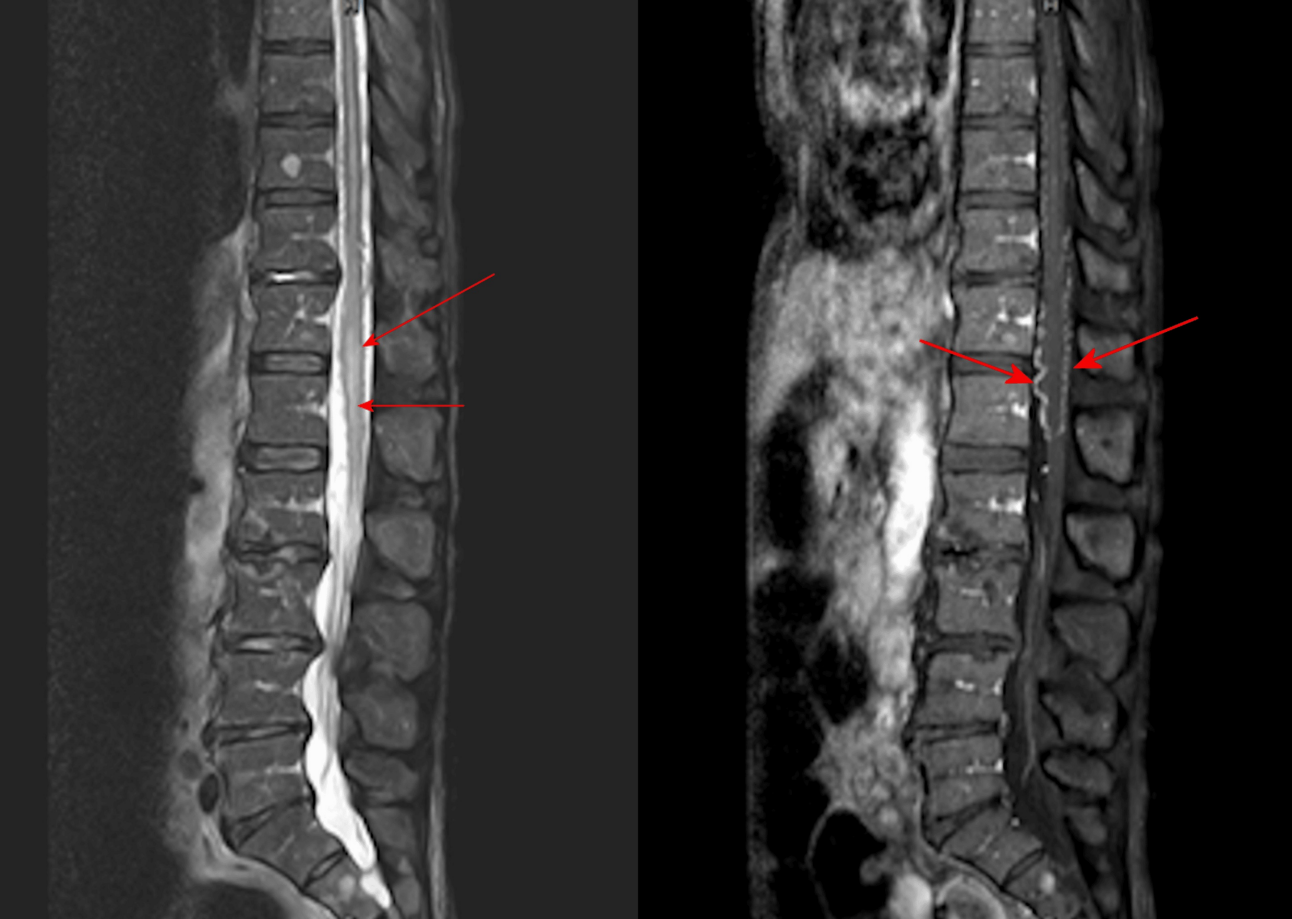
Myelopathy at T12-L1 under thoracolumbar spine magnetic resonance imaging (MRI) Coronal T2-weighted MRI of the thoracolumbar spine showing longitudinal spinal cord edema (red arrows), suggesting acute infarction due to venous congestion resulting from a dural arteriovenous fistula.
